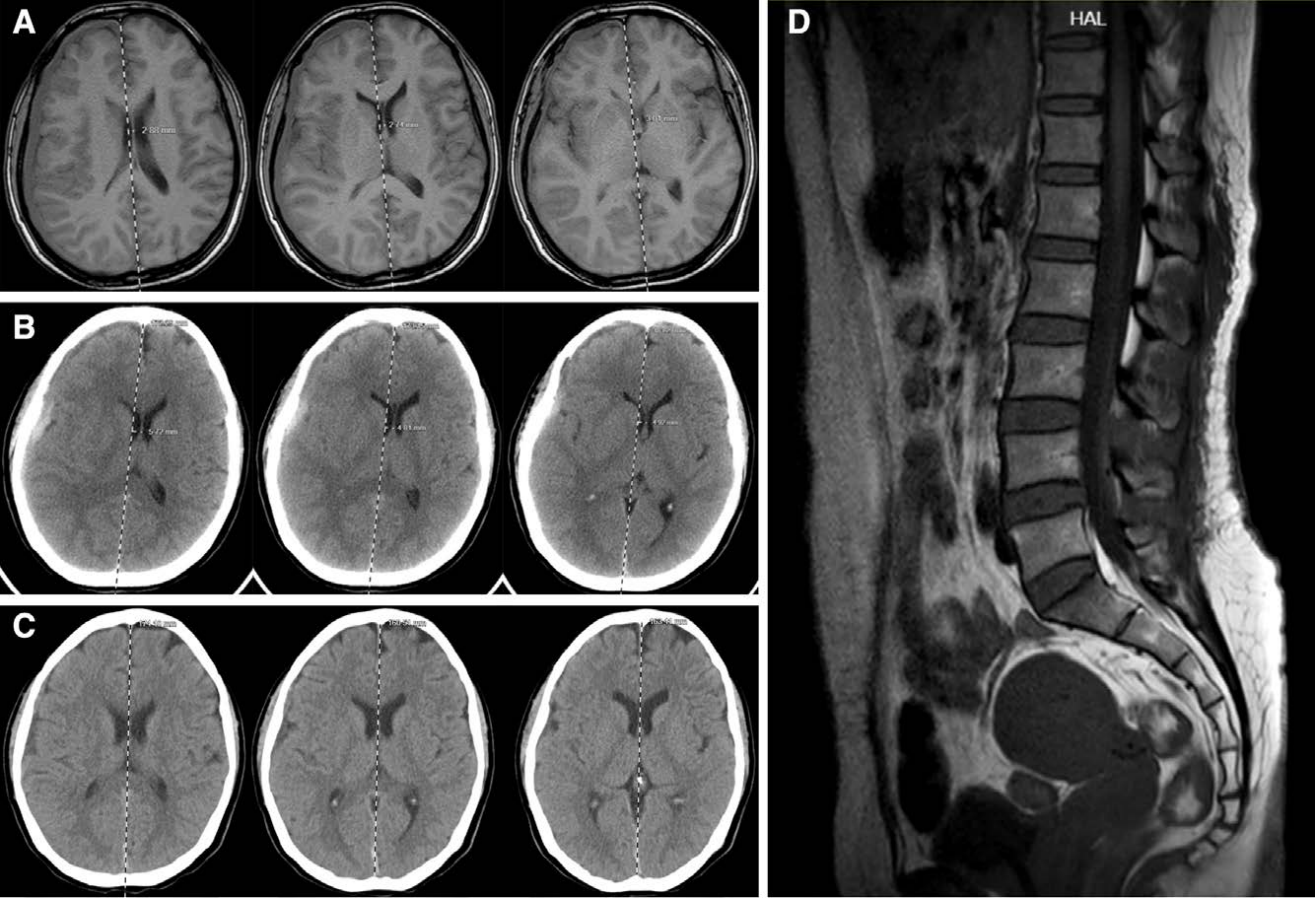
Postoperative imaging. (A) Admission MRI. (B) Postoperative Day 2 CT. (C) Postoperative CT. (D) Postoperative lumbar MRI. Compared to the Admission MRI, the Postoperative Day 2 CT demonstrated progressive midline shift. Postoperative CT demonstrated adequate evacuation of the hematoma with improvement in midline shift. Postoperative lumbar MRI demonstrated adequate evacuation of the spinal subdural hematoma. CT = computed tomography, MRI = magnetic resonance imaging.
